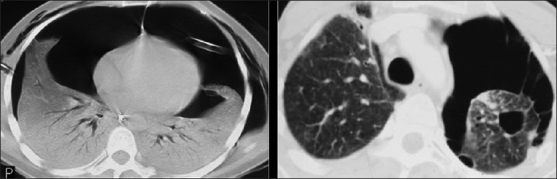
Pneumocystis carinii* pneumonia. Computed tomography showing bilateral pneumothoraces in conjunction with lung cysts
